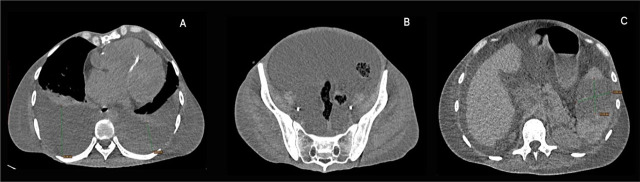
A. Chest CT scan: Large bilateral pleural effusions with atelectasis; Mild pectus excavatum with a Haller index of 2.28; B. Abdominopelvic CT scan: Large amount of ascites; C. Abdominal CT scan: Splenic infarcts
